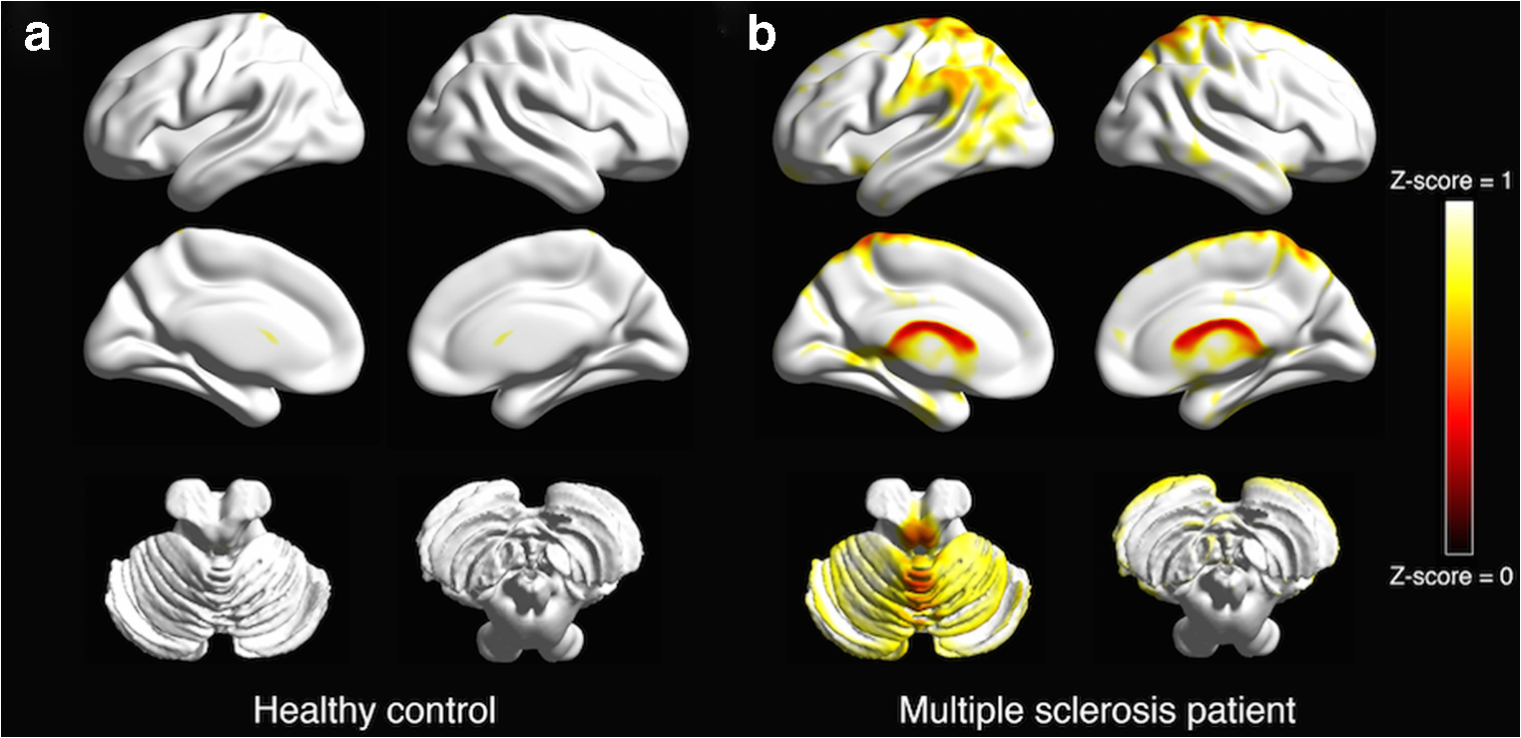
Mean [^18^F]florbetapir SUV_70–90_ in cortical grey matter. Cortical regions showing a mean SUV_70–90_ lower than the calculated cut-off for normal cortical myelination. (a) Healthy 38-year-old female and (b) 44-year-old female relapsing-remitting Multiple Sclerosis patient (disease duration = 18 years; Annualized-Relapse-Rate of 0.22; EDSS = 1; MSSS = 0.26). Cut-off was calculated as the mean of SUV_70–90_ in the cortical grey matter for healthy controls – 1.96 times standard deviation, which corresponds to the 5th percentile. Calculated cut-off was 1.0246. Images presented on the International Consortium for Brain Mapping (ICBM) whole brain surface visualized in BrainNet Viewer (http://www.nitrc.org/projects/bnv/); colour bar represents Z-scores
